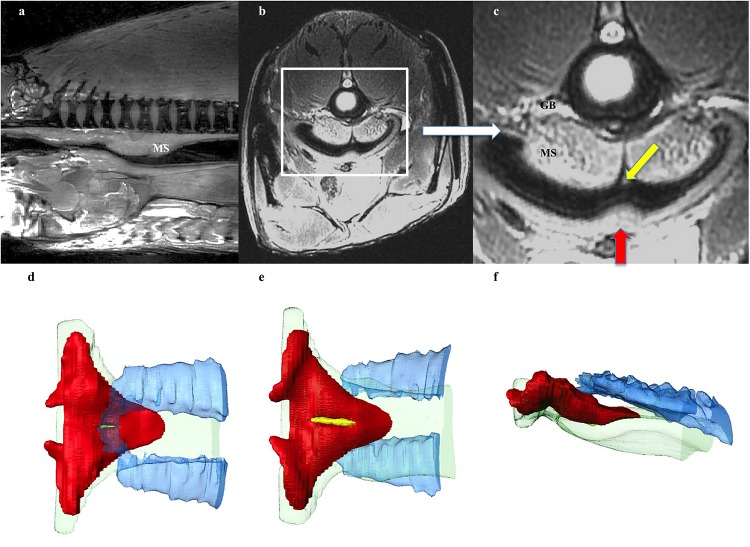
Respiratory system: Esophageal Sphincter. (a) Midline sagittal, and (b,c) axial MRI image at level of upper esophagus through the muscular sphincter (MS), positioned in the dorsal aspect of the esophagus. White box on (b) indicates enlarged section (c). The sphincter is a triangular, disc-like shaped muscle through which air moves between the esophagus and the rostral aspect of the gas bladder (GB), which is not inflated at this level. A tiny longitudinal strip of tissue seen overlying the opening (yellow arrow in c), may act as a valve regulating the flow of air, or preventing water/food from entering the GB. In addition a localized thickening of tissue (red arrow) sited on the opposing side of the esophagus, possibly occludes the slit opening when the surfaces of the esophagus are opposed. (d,e) 3D reconstruction of muscular sphincter from CT data demonstrating relationship between the esophagus and the GB. Red: muscular sphincter, blue: rostral end of GB, green: esophagus, yellow: strip of tissue in valve opening. (d) From above sphincter, (e) from below sphincter, (f) lateral view.
